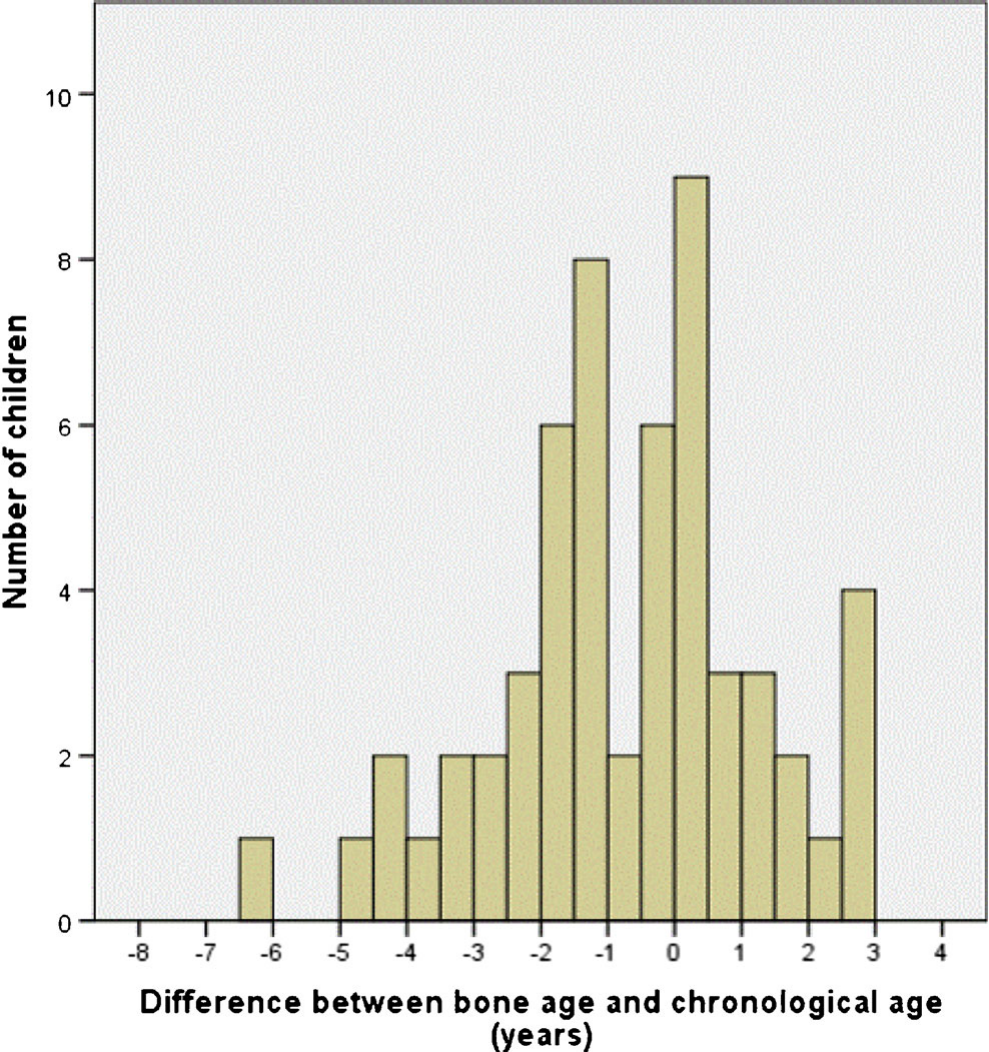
Histogram shows differences between chronological age and bone age (*n* = 56). Chronological age is defined as age in years at time of measurement. Bone age is the automated bone age in years as determined by BoneXpert software based on hand radiographs
